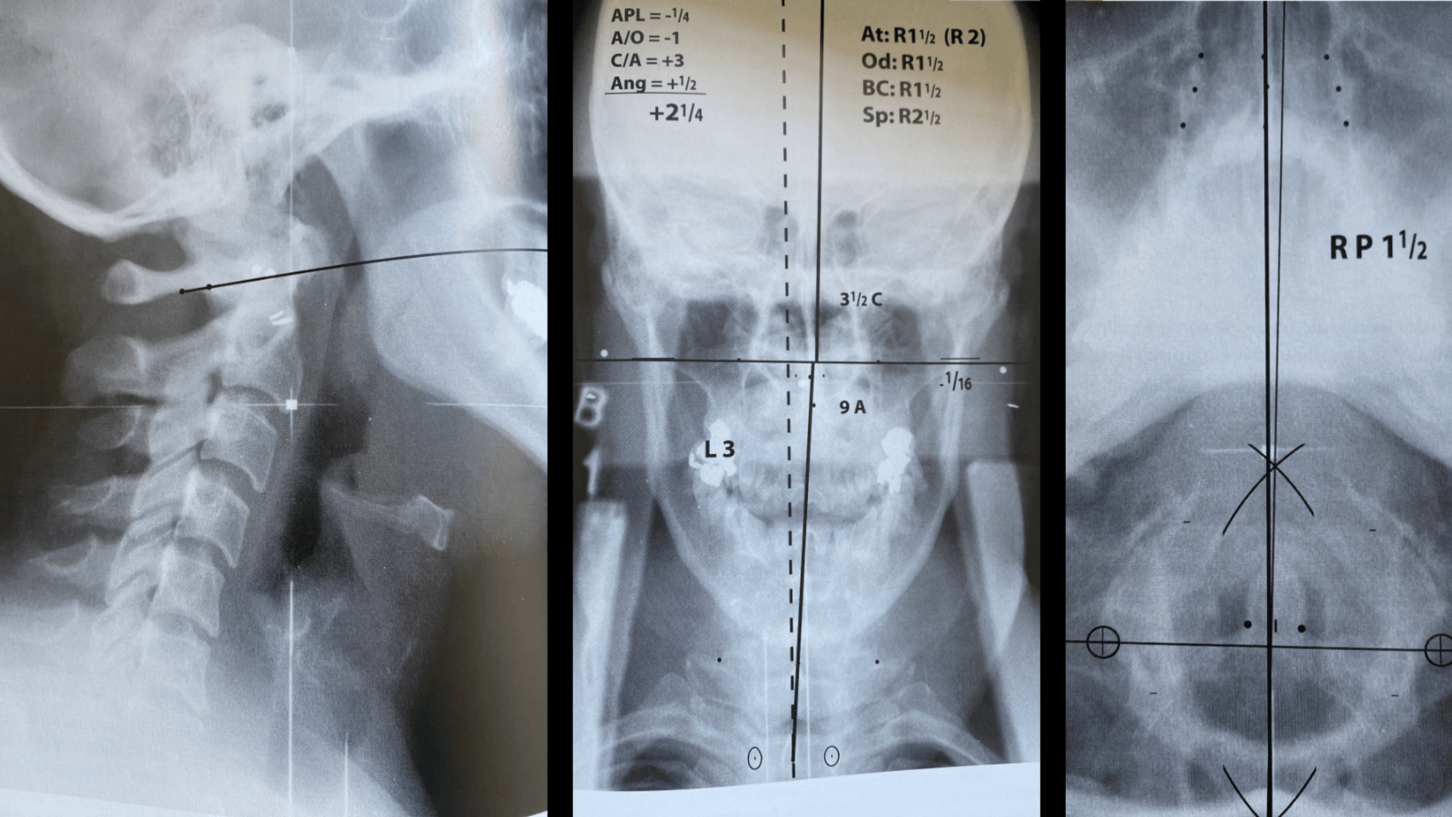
NUCCA radiographs and analysis examples Lateral cervical (left), nasium (middle), and vertex (right) Courtesy of and published in *NUCCA Protocols and Perspectives: A Textbook for the National Upper Cervical Chiropractic Association* [16]. Permission was obtained for use in this article. NUCCA: National Upper Cervical Chiropractic Association
